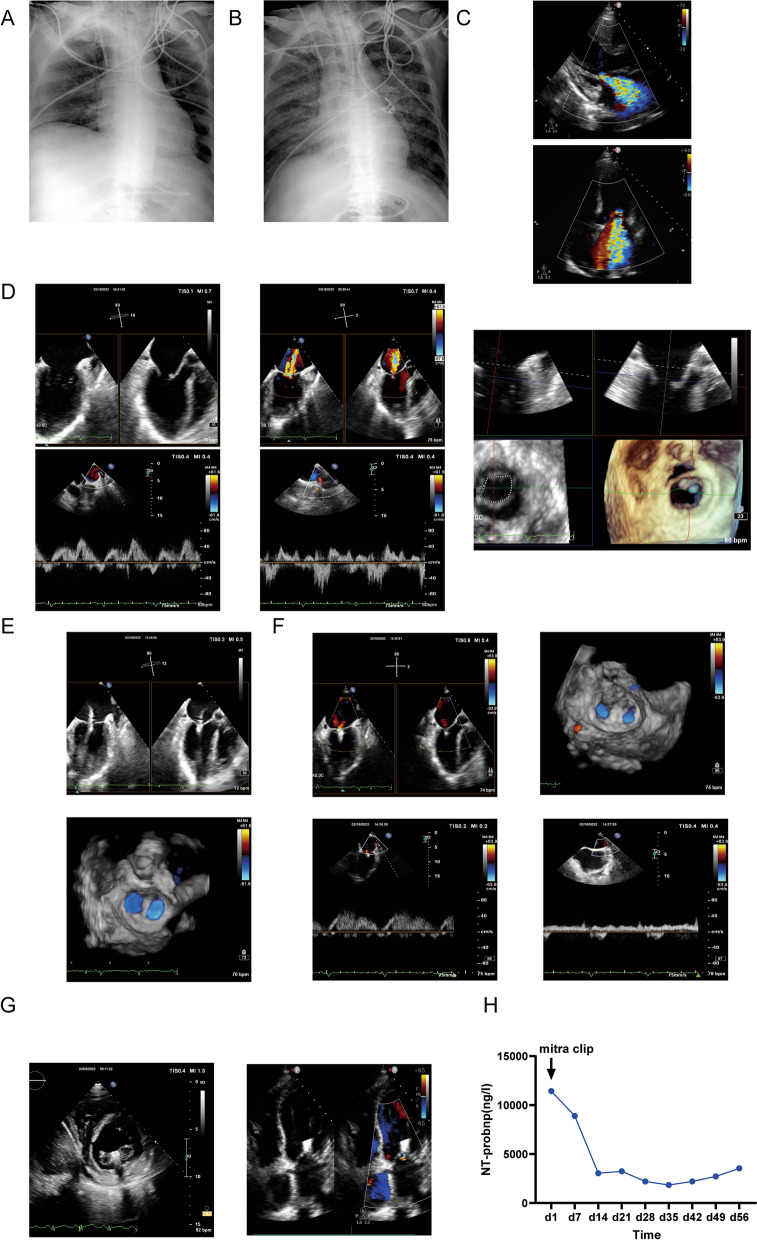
A Chest radiography at admission; B Chest radiography for review; C Cardiac ultrasound before operation; D Cardiac esophageal ultrasound and pulmonary venous flow pattern before operation; E Cardiac esophageal ultrasound after the first clip release. F Cardiac esophageal ultrasound and pulmonary venous flow pattern after the second clip release. G Cardiac ultrasound after operation; H NT-proBNP after operation
